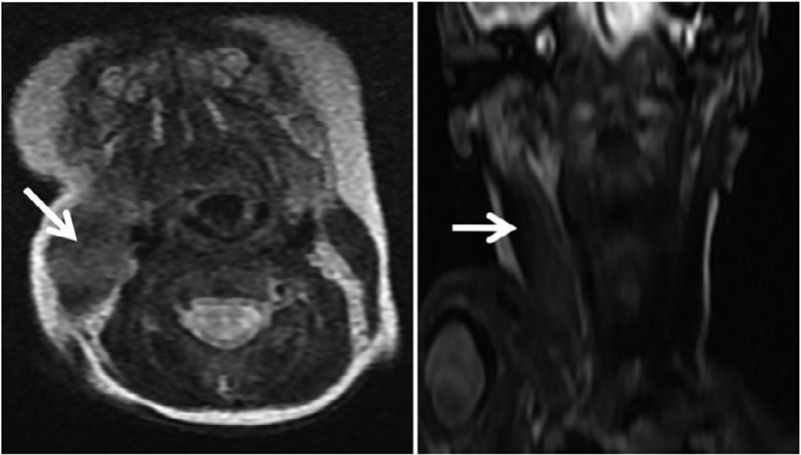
MRI T2 axial and coronal sequence showing the fusiform hypertrophy of the right sternocleidomastoid muscle in hypersignal (white arrow) compared to the left one.
